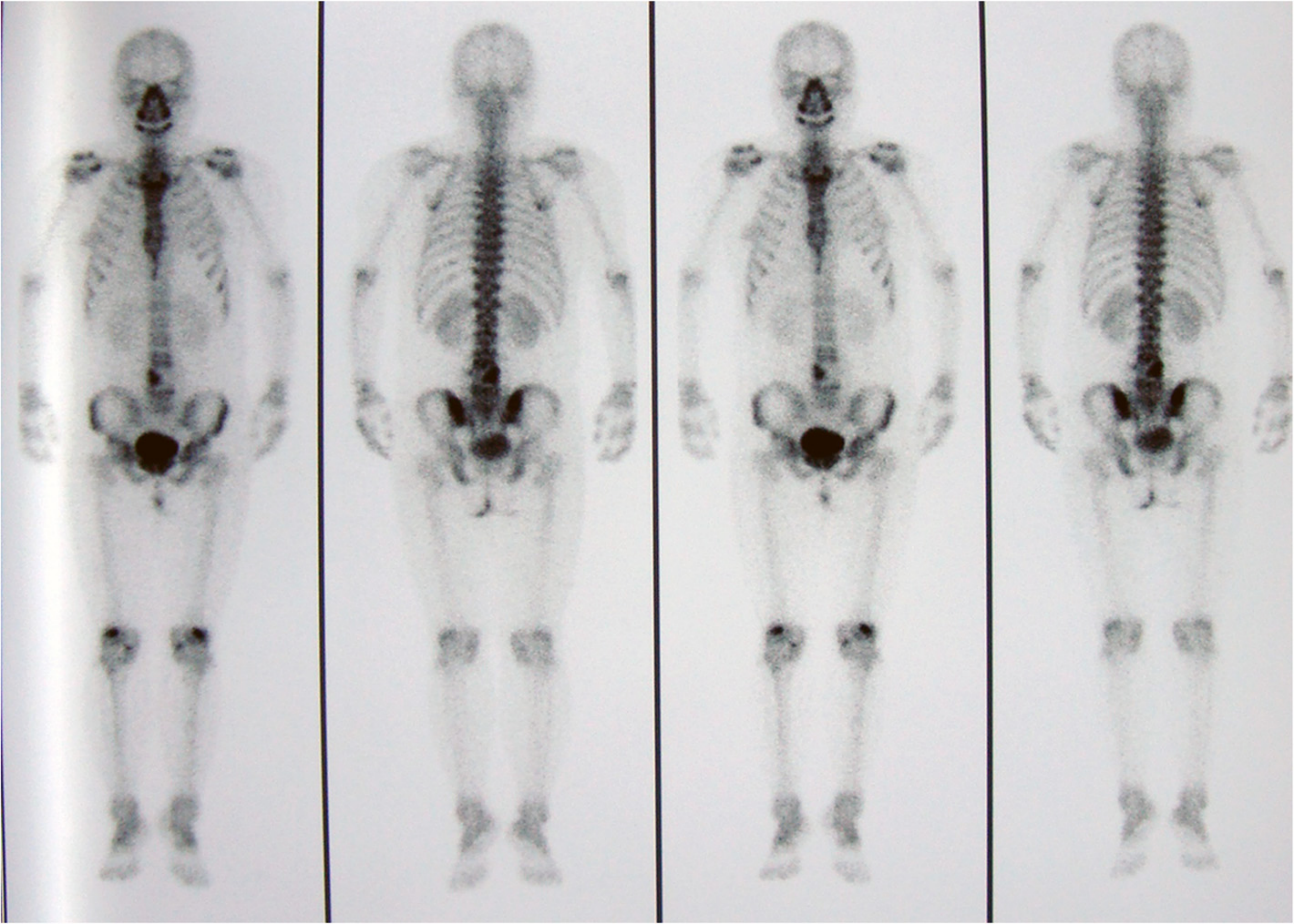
SPECT examinations. Increased uptake of technetium-99m in the area of L4 vertebrae.
